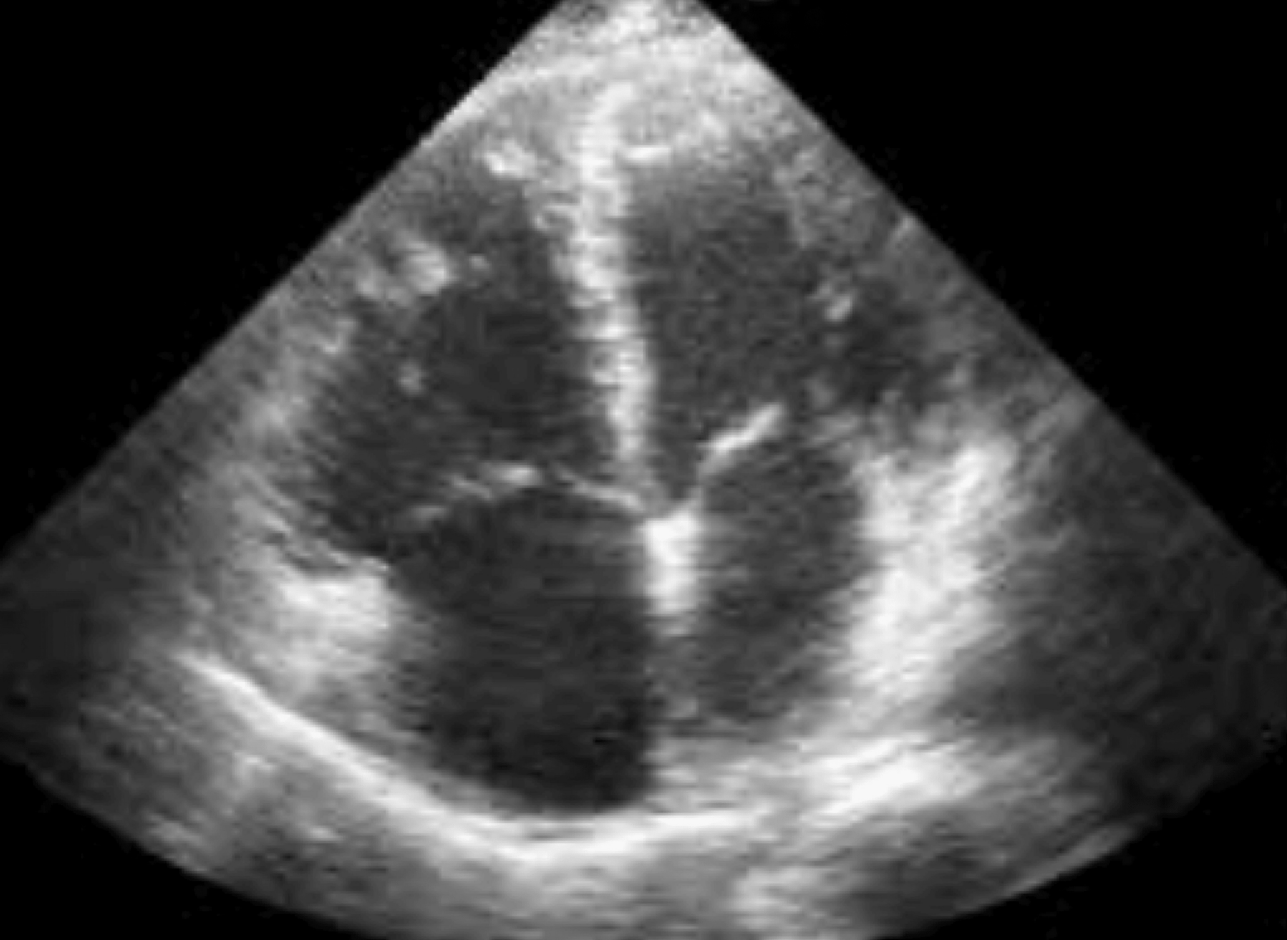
No signs of malformation were noted on transthoracic echocardiography
